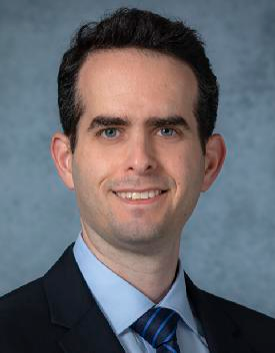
Dr Alon Shechter, MD MHA FESC graduated from the Tel Aviv University School of Medicine, and completed both his internal medicine residency and general cardiology fellowship at Rabin Medical Center. He later completed a clinical echocardiography fellowship at Rabin and a research fellowship at Cedars-Sinai Medical Center. Currently, Dr Shechter serves as a staff cardiologist at Rabin's echo lab and valve clinic. His research focuses on valvular heart disease and structural heart interventions.
